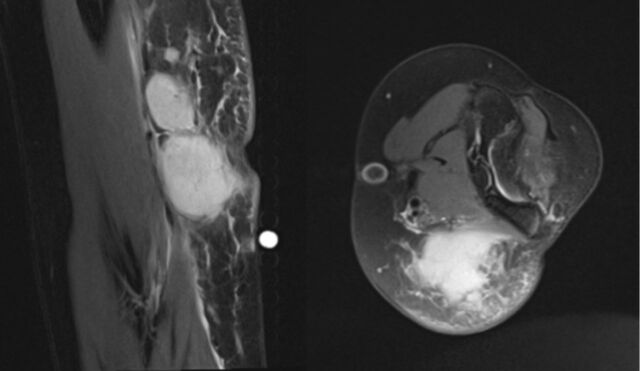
T2-weighted magnetic resonance imaging of the left elbow displays abscess formation.
